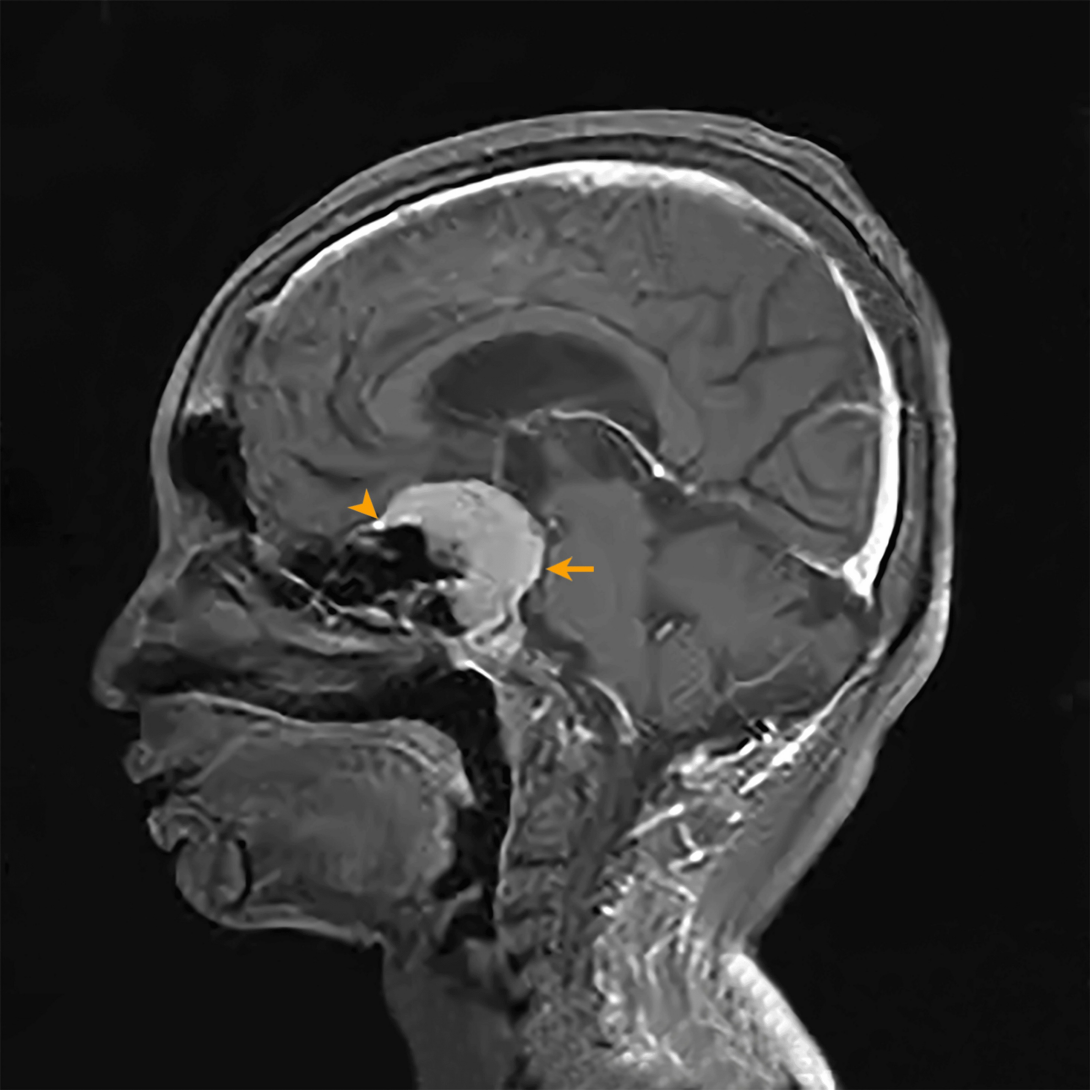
MRI gadolinium-enhanced T1-weighted sagittal image. A well-defined enhancing lesion of 4.3 x 3.9 x 3.6 cm in the suprasellar extending into the sella (orange arrow). The lesion shows a dural tail sign (orange arrowhead), suggesting a meningioma. There is no separate visualization of the pituitary gland MRI: magnetic resonance imaging
